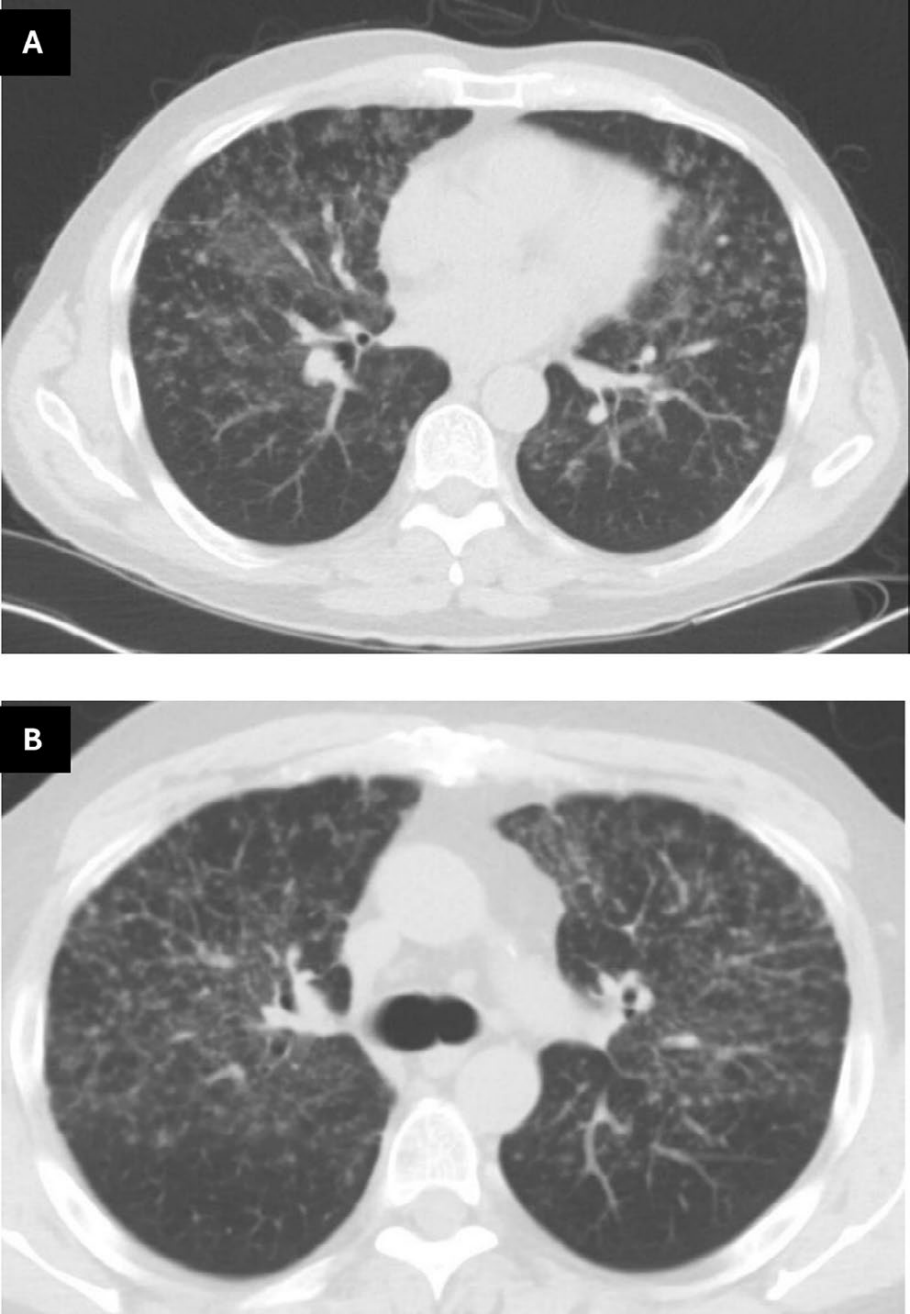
Chest CT with diffuse upper lung predominant perilymphatic micronodules.
